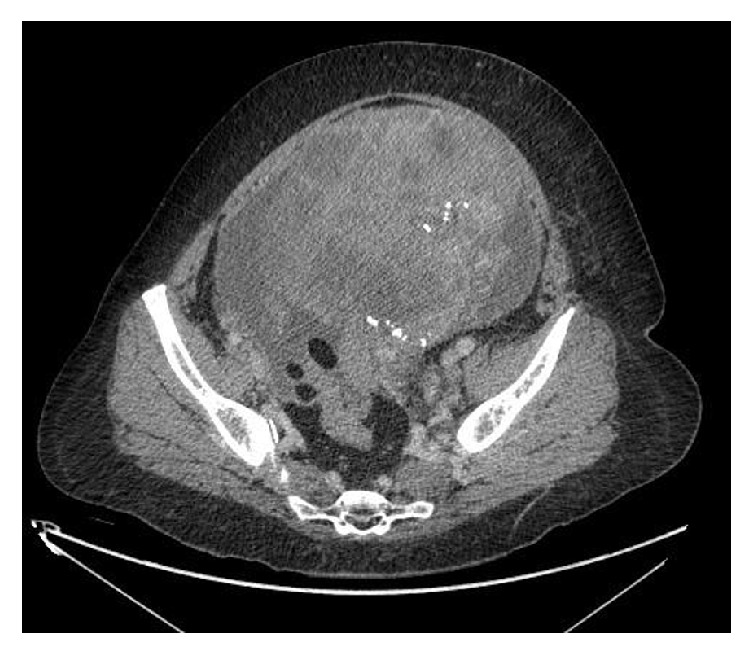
CT scan of the abdomen showing a large heterogeneous, partially necrotic abdominal-pelvic mass, 16.3 cm by 20.2 cm in size.
